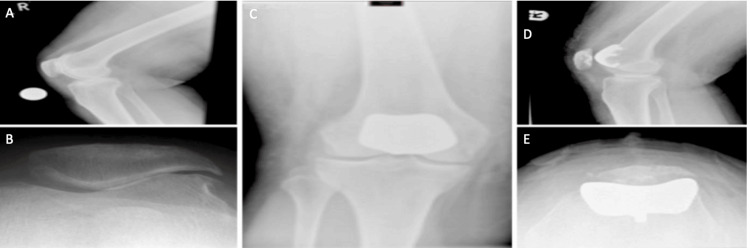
Pre-op and post-op PFJR with Journey prosthesis (A) Pre-operative lateral view knee X-ray (B) Pre-operative skyline view knee X-ray (C) Post-operative AP weight-bearing view knee X-ray with Journey prosthesis (D) Post-operative lateral view knee X-ray with Journey prosthesis (E) Post-operative skyline view knee X-ray with Journey prosthesis PFJR: patellofemoral joint replacement; AP: anteroposterior
